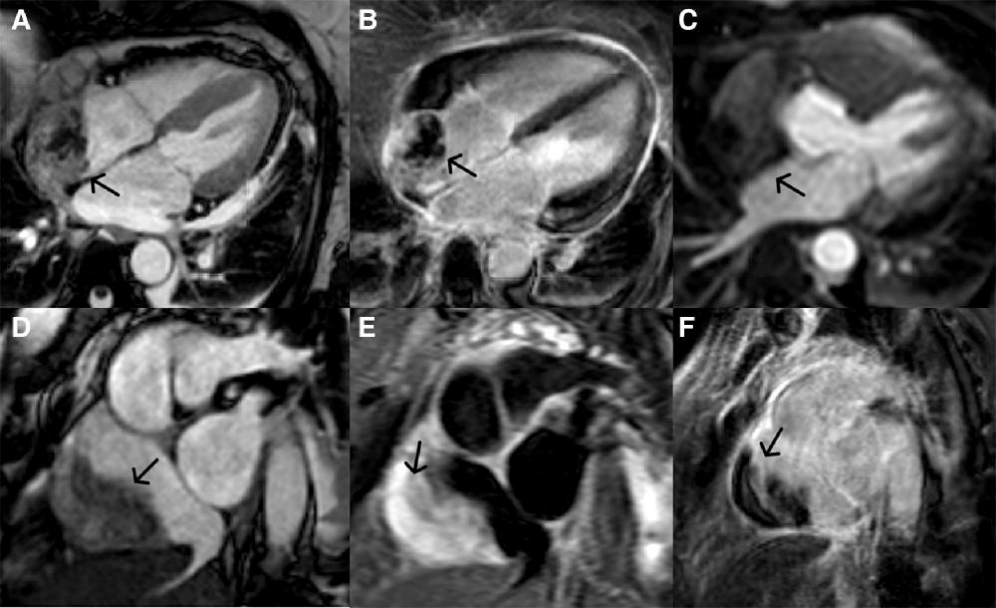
Cardiac MRI demonstrating a mass in the wall of the right atrium with suspected pericardial infiltration in cine imaging (bFFE; four-chamber view). (*A*) The following late enhancement sequence (SPIR; four-chamber view) is showing peripherally accentuated, heterogeneous late enhancement in the lesion. Furthermore contrast enhancement (gadolinium) of the pericardium can be observed, as well as subepicardial enhancement (midventricular lateral). (*B*) Perfusion images, showing no contrast enhancement in the mass and remaining myocardium. (*C*) Short axis view in cine imaging (bFFE) (*D*), T2W-STIR imaging (showing a hyperintense lesion) (*E*) and LGE imaging for further characterization of the mass in different views (*F*).
